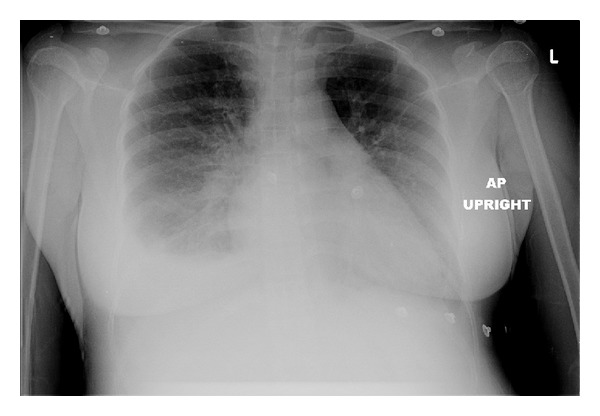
Chest X-ray showing bilateral pleural effusion and pulmonary congestion on admission.
